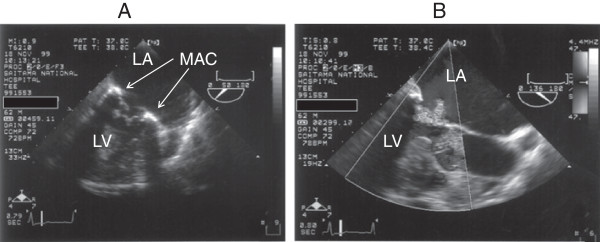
(A) Preoperative transesophageal echocardiogram revealed serious mitral annular calcification. (B) Doppler echocardiogram revealed severe mitral regurgitation due to chordae rupture of the posterior medial scallop. MAC: mitral annular calcification; LA: left atrium; LV: left ventricle.
